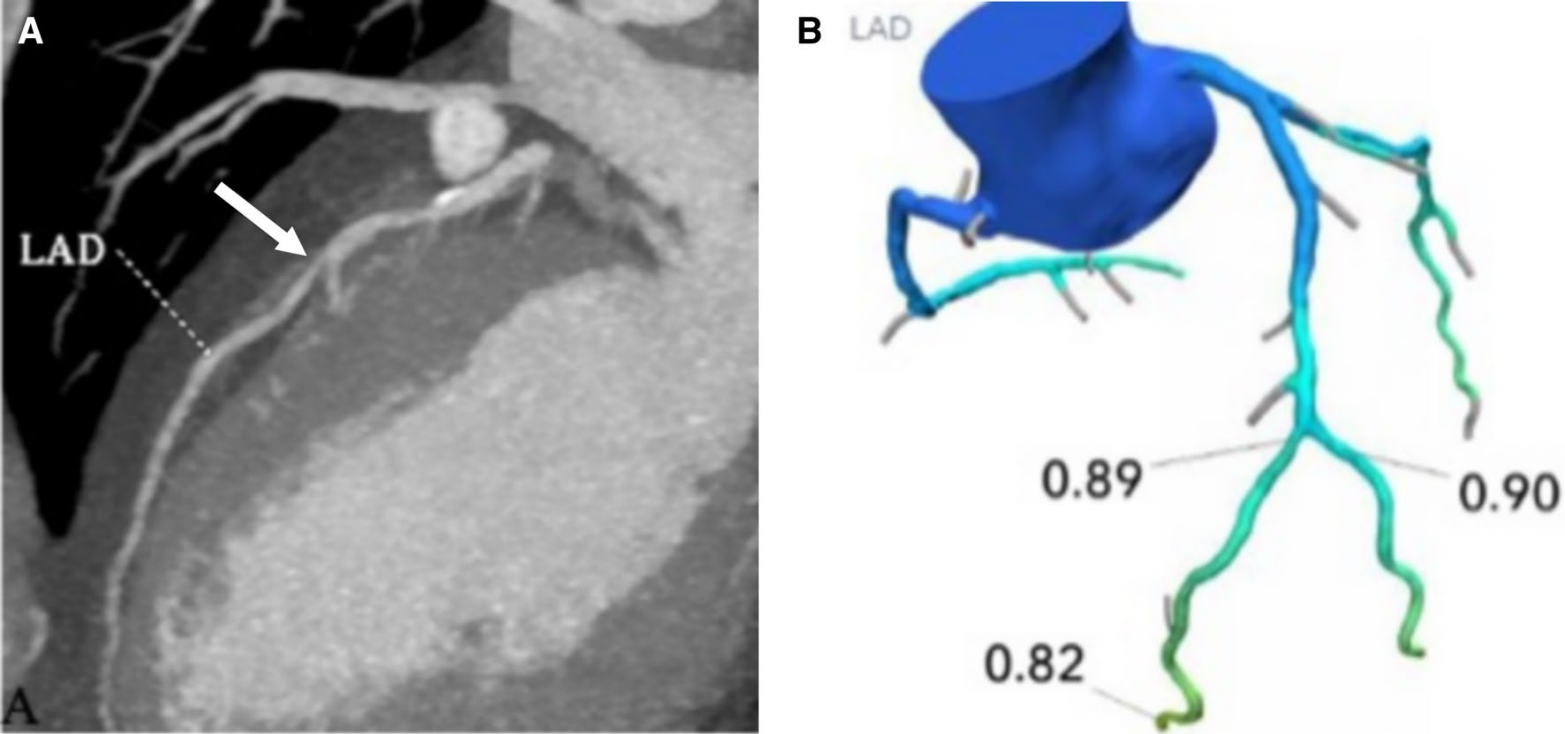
a A curved multiplanar reconstruction image of the LAD in a 52-year-old man with chest pain showing moderate stenosis of the mid LAD (arrow). b CT-FFR in the same patient shows a value of 0.89 in the mid LAD, which is within normal limits, indicating that there is no lesion-specific ischemia. The patient was referred for medical management. Therefore, CT-FFR helped to avoid ICA. *CT* computed tomography, *FFR* fractional flow reserve, *ICA* invasive coronary angiography, *LAD* left anterior descending artery
